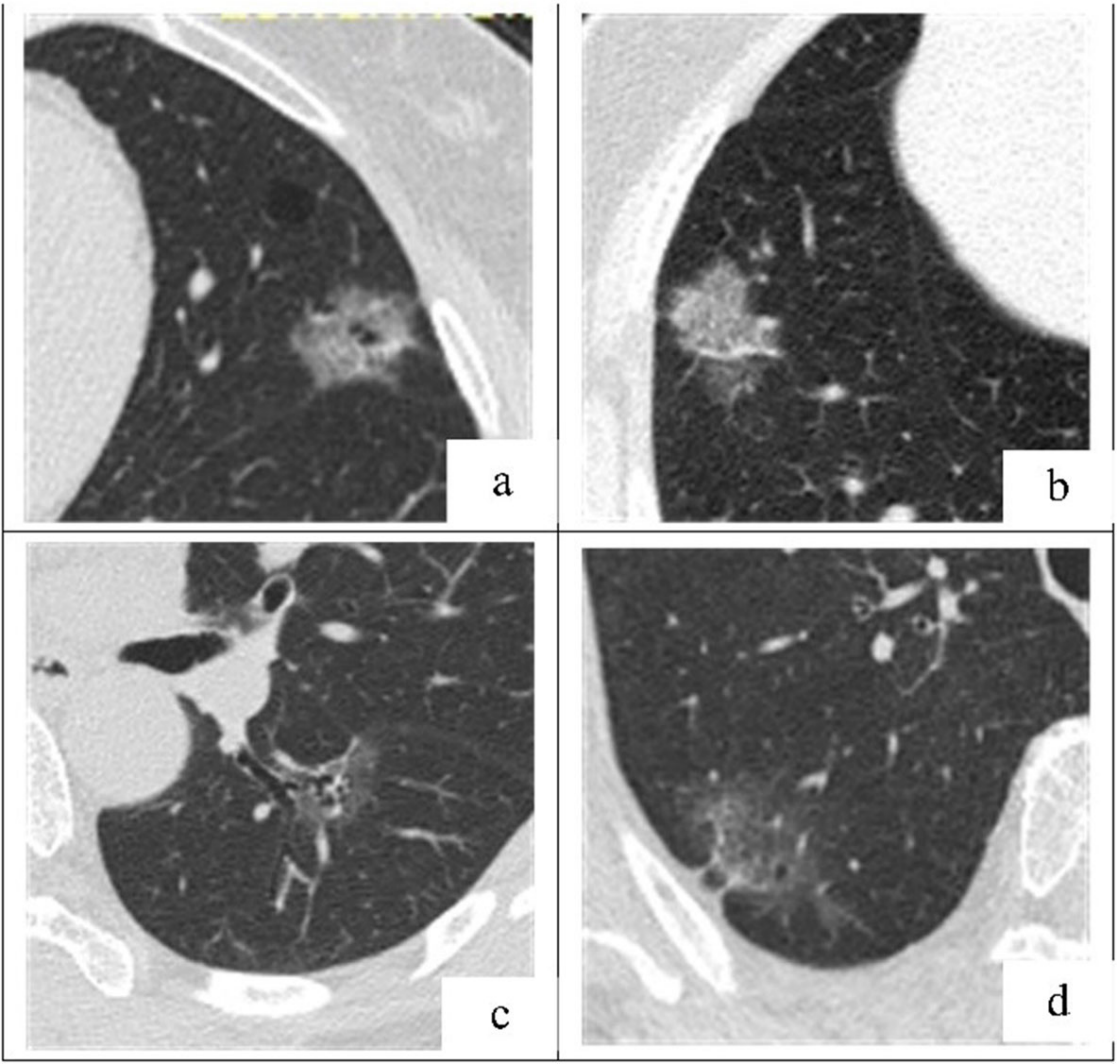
a-d. Representative axial TSCT images of P-pGGNs in four patients, connected to the visceral pleural surface. The longer TLD, higher CT-LAP, lobulation signs and bubblelike-appearance suggest P-pGGN invasiveness. a A 63-year-old female with a P-pGGN in the left superior lobe, diagnosed as IAC, showed a 2.32 cm-TLD, lobulated, bubblelike-appearance, and − 467.31 HU-CT-LAP; b A 59-year-old female with a P-pGGN in the right superior lobe, diagnosed as IAC, showed a 3.09 cm-TLD lobulated and − 360.13 HU-CT-LAP; c A 57-year-old female with a P-pGGN in the left inferior lobe, diagnosed as MIA, showed a 2.36 cm-TLD, and − 630.43 HU-CT-LAP; d An 80-year-old female with a P-pGGN in the right superior lobe, diagnosed as MIA, showed a 4.16 cm-TLD, and − 662.7 HU-CT-LAP
